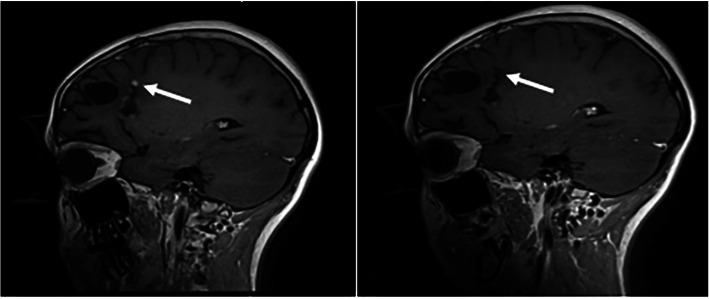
Representative T1 postcontrast MRI images of a pediatric *NTRK*-fused glioma treated with targeted TRK inhibitor therapy. (a) Recurrent/residual tumor along the patient’s resection cavity (arrow) demonstrated (b) radiologic response to larotrectinib (arrow)
